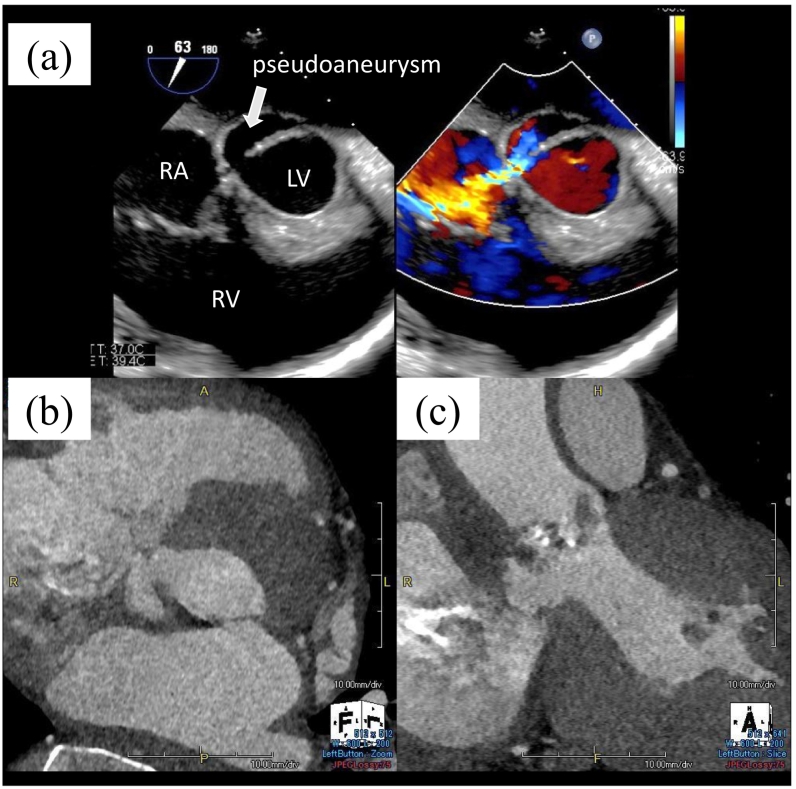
Preoperative transesophageal echocardiography and computed tomography images. An abnormal cavity around the aortic root leading to the left ventricle and right atrium, with the thickened wall (a) (LV: left ventricle, RA: right atrium, RV: right ventricle). The left ventricular outflow tract pseudoaneurysm penetrated the right atrium without pericardial effusion (b: axial, c: coronal).
